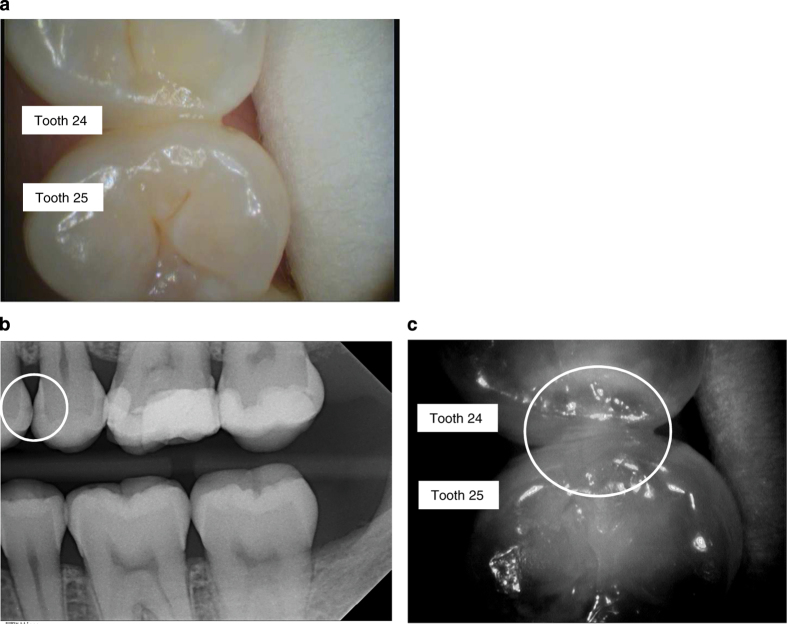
Examples of images using the VistaCam iX Proxi head. (a) Image of teeth with the intraoral camera (24 distal, 25 mesial). (b) Corresponding bitewing radiograph (D2 lesion seen in 24 distal, D3 lesion seen in 25 mesial, this surface was not included in the study). (c) Corresponding NIR image. The circle indicates the area that is associated with demineralisation of the enamel.
